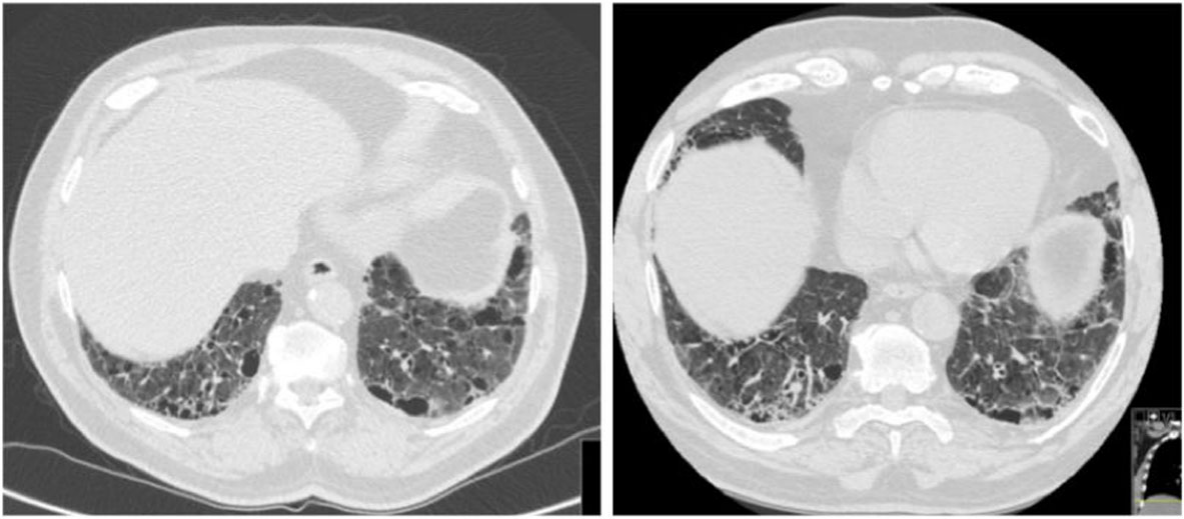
HRCT of CPFE patient. Baseline (left) and during AE-COPD (right). Findings include peribronchial wall thickening; note absence of GGOs
